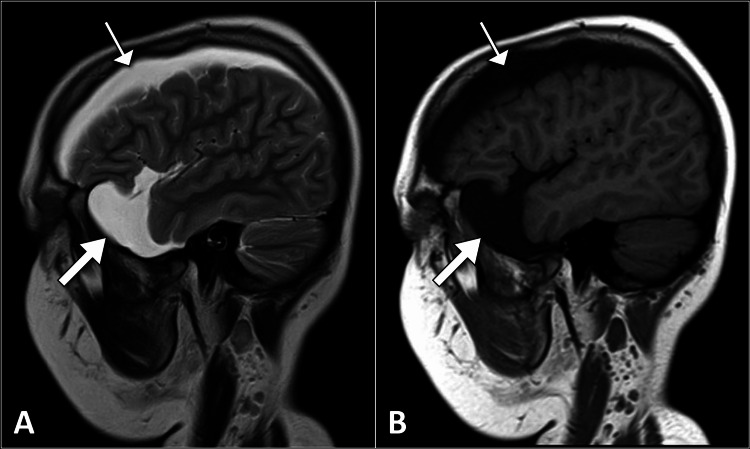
Sagittal brain MRI images show a cystic lesion in the temporal region (thick arrow) with extension along the cerebral convexity (thin arrow), which demonstrates a high signal on the T2-weighted image (A) and a low signal on the T1-weighted image (B) MRI: magnetic resonance imaging
